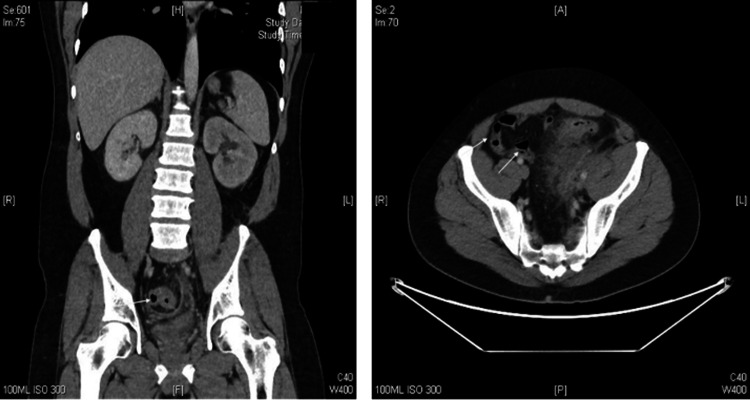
Computed tomography scan (coronal and axial planes) demonstrating microperforation (arrows)
